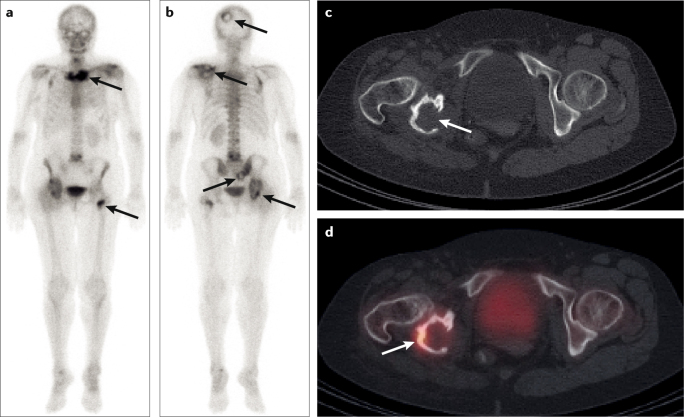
Radionuclide pattern of bone metastases from RCC. Multiple osseous bone metastases (arrows) detected by technetium-99 m (^99m^Tc)-phosphonate bone scintigraphy in anterior (part a) and posterior (part b) views. Transversal CT (part c) depicts osteolysis of the posterior right acetabulum (arrow), which shows only partially activated bone metabolism (arrow) on single-photon emission CT (SPECT)–CT (part d). RCC, renal cell carcinoma.
